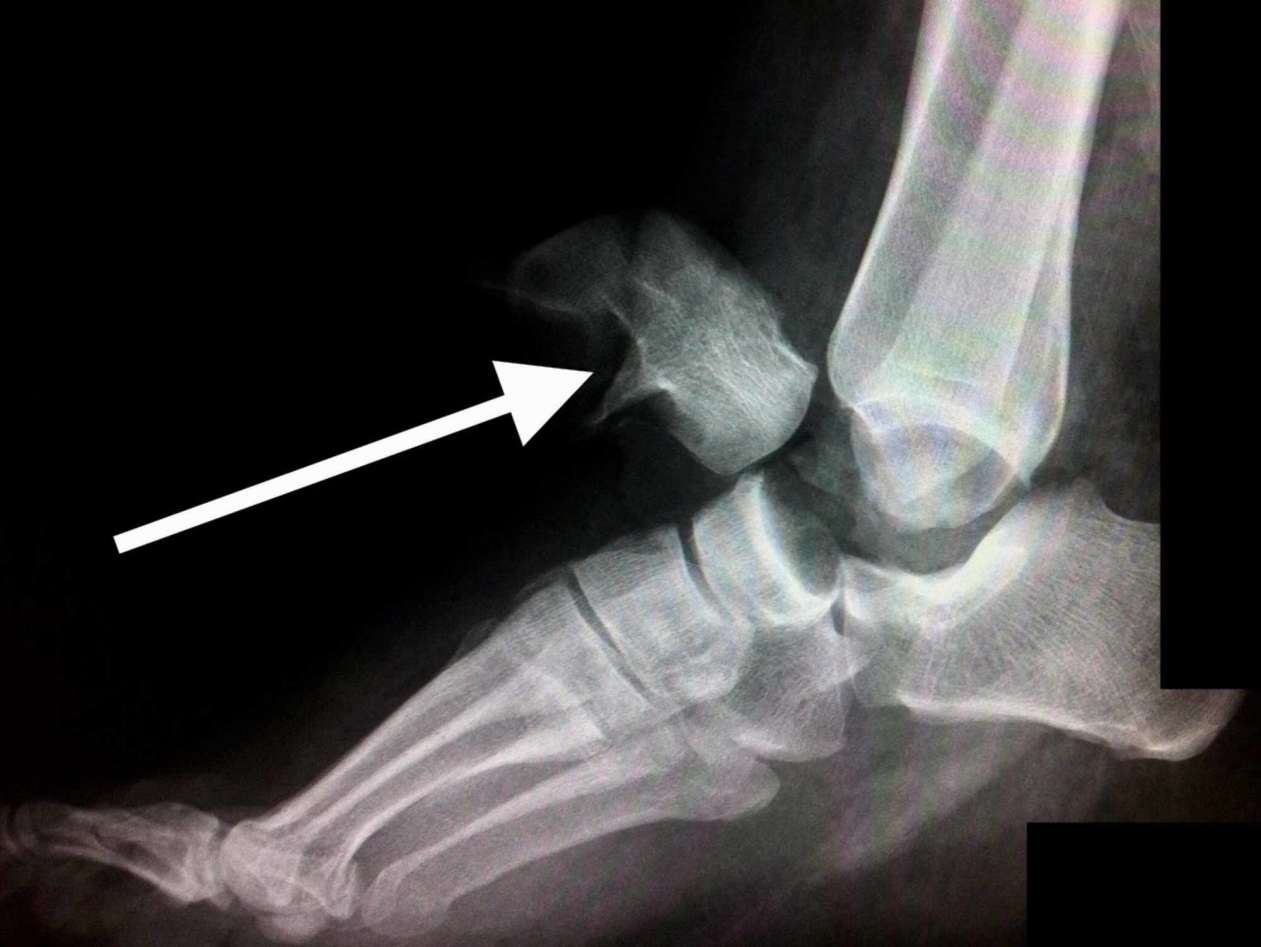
Preoperative lateral radiograph of the right ankle demonstrating pan-talar dislocation (arrow)
